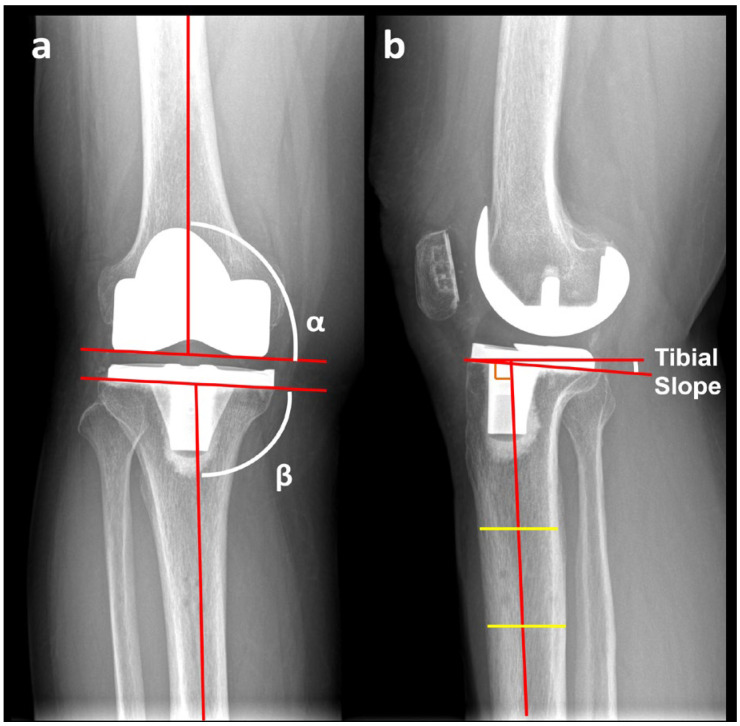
Radiographic measurements of component alignment following total knee arthroplasty. (a) Coronal plane: α = femoral component angle, and β = tibial component angle. (b) Sagittal plane: tibial slope angle measured between the tibial baseplate and the line perpendicular to the tibial anatomic axis (two-point method).
